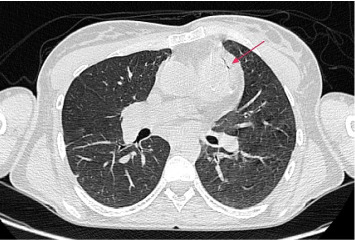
Cardiac CT scan shows fluid collection surrounded by a thick layer of inflammatory-enhancing tissue as perivalvular abscess (red arrow).
